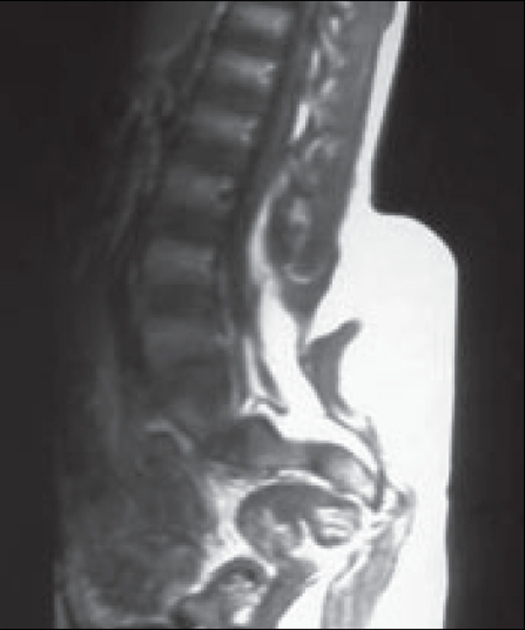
Saggittal T1W MRI showing large lipoma extending subcutaneously through the bony defect. Segment of the rudimentary accessory limb also seen
